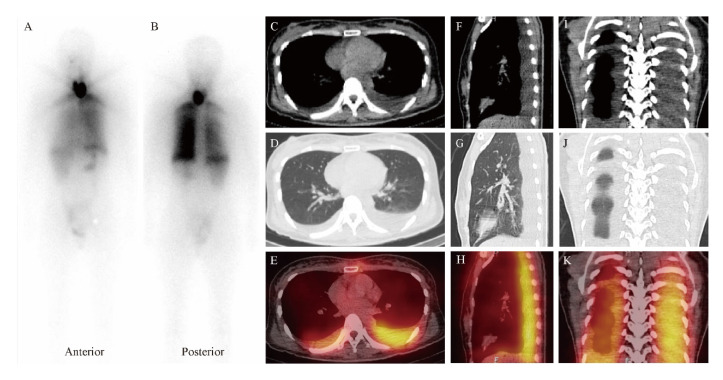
Whole-body scan ((A), anterior view; (B), posterior view) on day 3 post ^131^I administration, showing ^131^I distribution in left thyroid lobe and chest. SPECT/CT images of chest ((C–E), transaxial; (F–H), sagittal; and (I–K), coronal) showing bilateral pleural effusion, predominantly in the left side. Neither abnormal uptake nor lesion was found in either lung.
